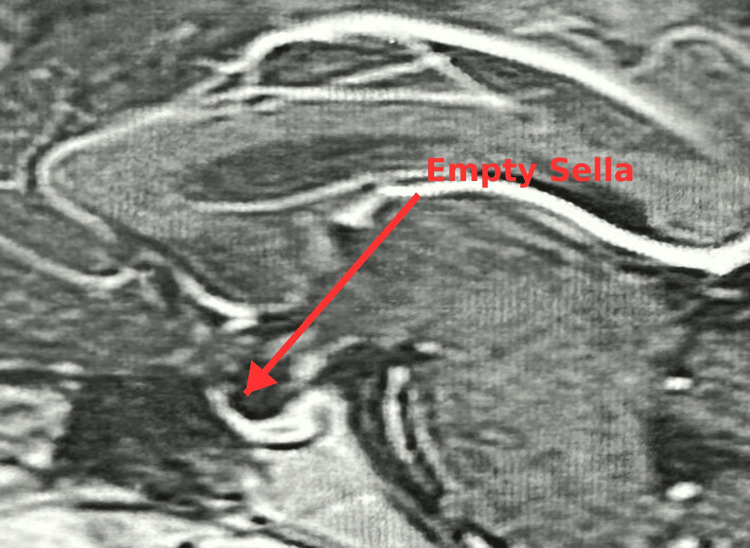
Pituitary MRI (sagittal T1-weighted view) demonstrating partial empty sella syndrome. Pituitary MRI, sagittal T1-weighted view, demonstrating partial empty sella syndrome. The red arrow indicates the region of cerebrospinal fluid herniation into the sella turcica, with flattening and thinning of the anterior pituitary gland against the sellar floor. The posterior pituitary bright spot and the pituitary infundibulum are preserved and in a normal midline position. No focal intrasellar or parasellar lesion, microadenoma, or suprasellar extension was identified. These features are characteristic of a partial primary empty sella, with no associated structural pituitary pathology.
